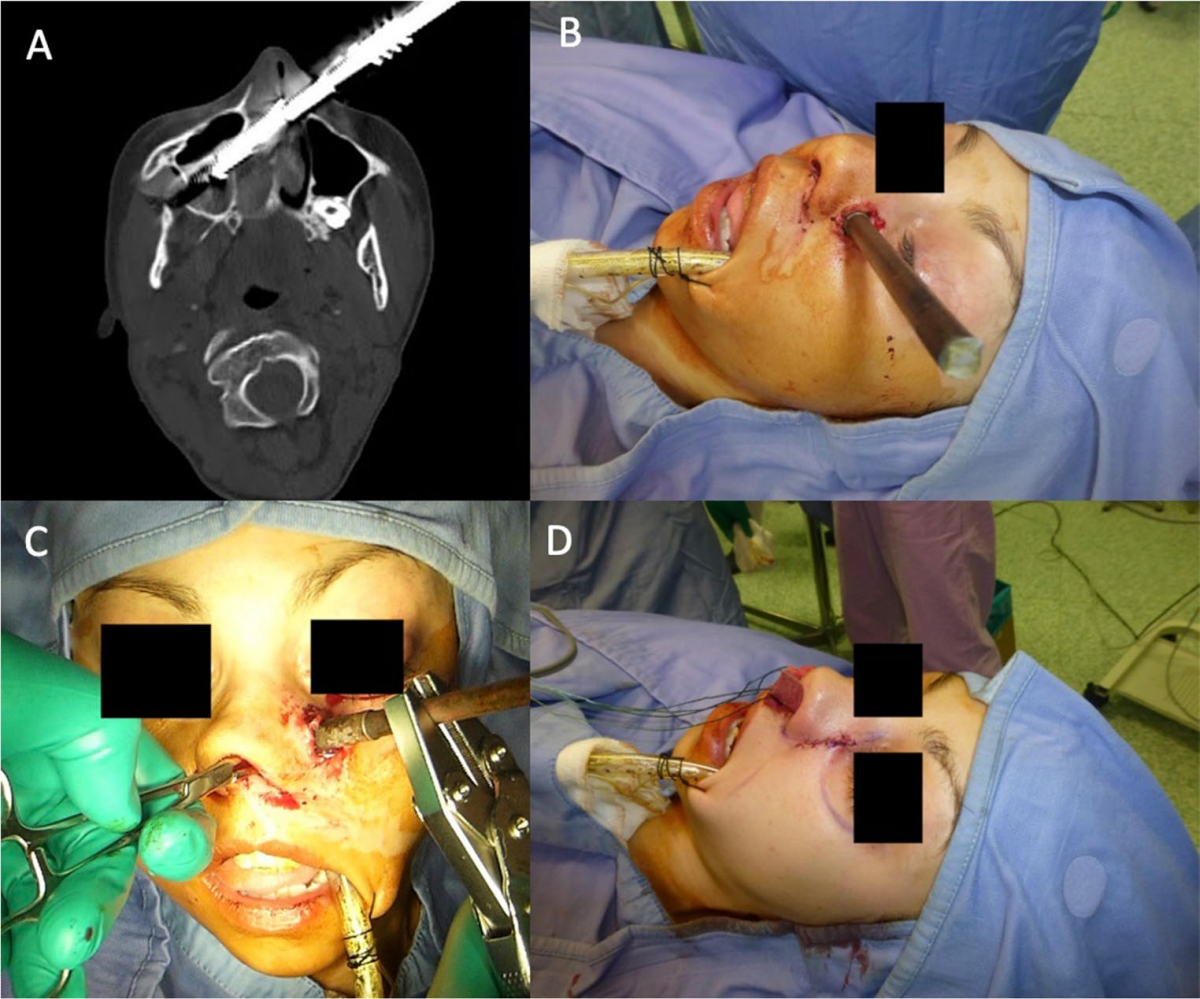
Case 1 – craniofacial speargun injury. (A) Axial CT scan showing the spear entering the left paralateral nasal region, crossing both nasal cavities and lodging in the medial wall of the right maxillary sinus. (B) Preoperative clinical view with the shaft protruding from the left paranasal area. (C) Intraoperative view of controlled retrograde extraction, with a Kocher clamp applied to the barb. (D) Immediate postoperative appearance after layered wound closure and bilateral anterior nasal packing
